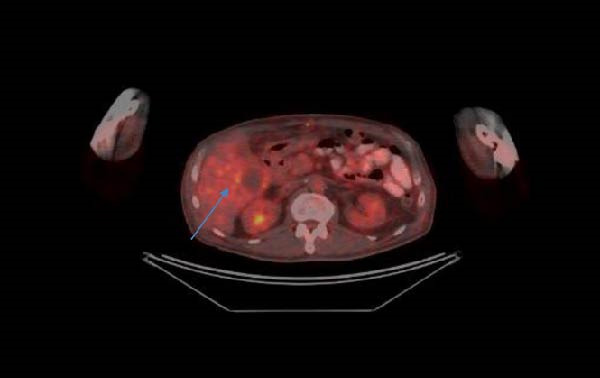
PET/CT data of metabolic activity in the liver.
